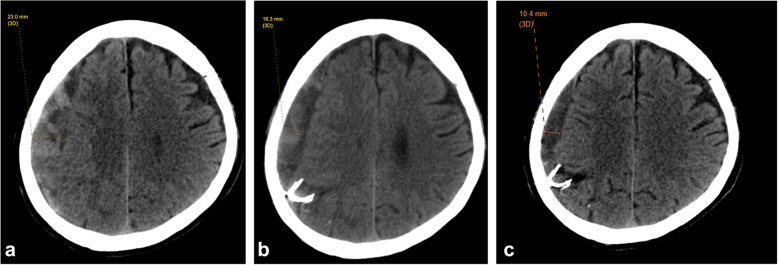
Serial CT brain selected images of the patient. a baseline b 1 month post MMA embolization and c 3 months post MMA embolization. There is gradual reduction in the thickness of the right chronic subdural haematoma on serial CT brain. Slender and C-shaped Onyx cast is seen at the posterior right parietal region with small area of infarct on 1 and 3 months post embolization CT brain images
